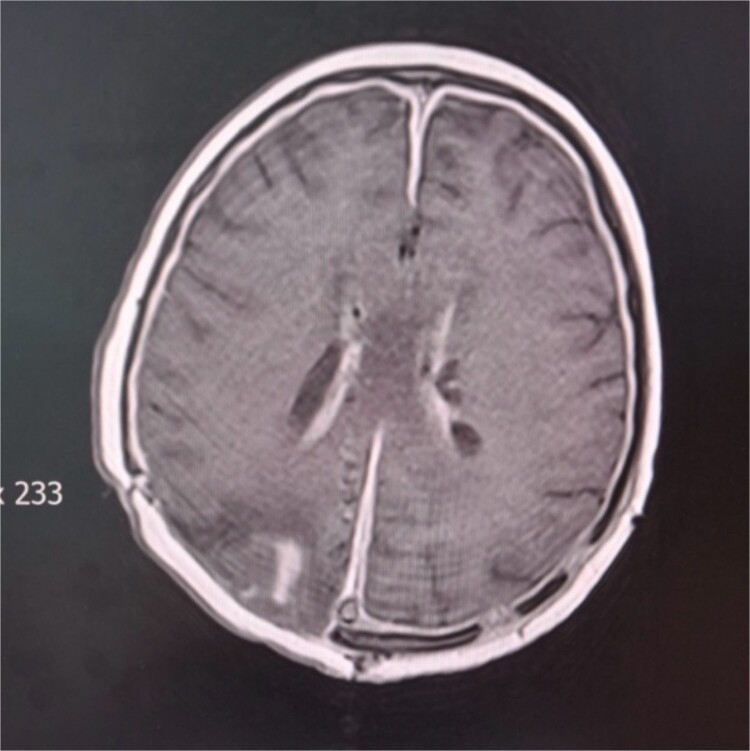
Cerebral MRI. Axial T1 weighted MRI showing ventriculitis with two external ventricular drainages (EVD) in the frontal horns of lateral ventricles.
